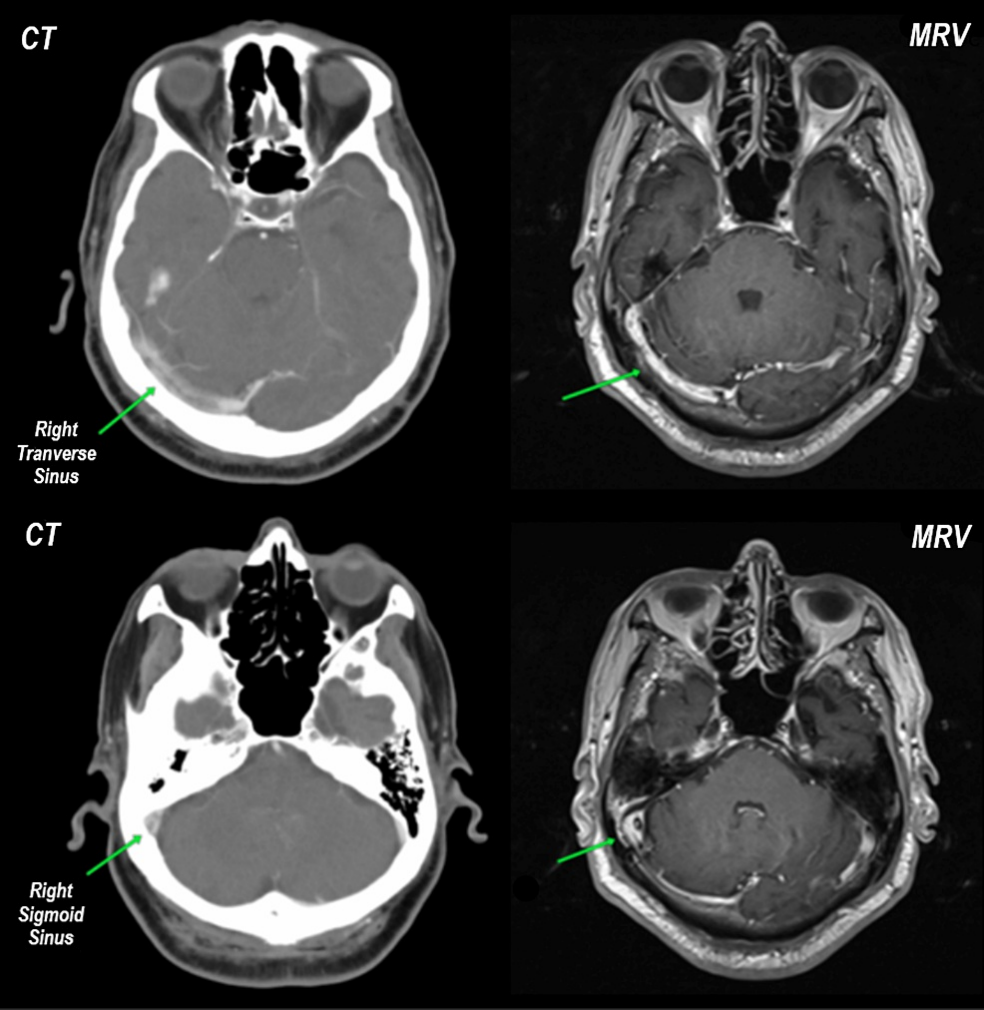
Contrast-enhanced computed tomography (CT) and magnetic resonance venography (MRV) of the brain in a post-gadolinium T1-weighted sequence showed a central linear irregular filling defect of the right transverse sinus extending to the sigmoid sinus. The filling defect is thick and has an irregular outline with peripheral enhancement suggestive of incomplete recanalization of chronic dural venous sinus thrombosis.
